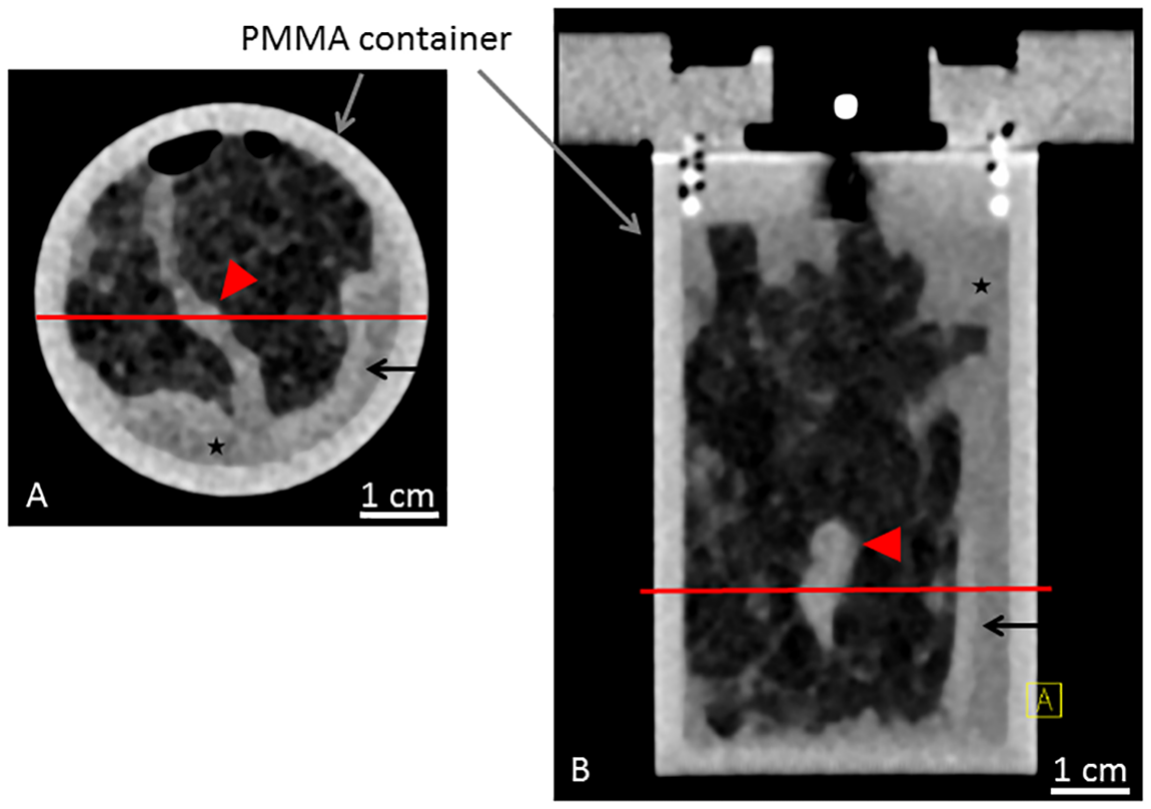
Clinical CT scan of sample B. Clinical CT scan of sample B in the sample holder with a standard clinical whole body CT scanner (Somatom Definition Flash, Siemens, Germany). Axial (A) and coronal (B) view. Red line in (A) and (B) reference for the respective imaging position. Asterisk indicating formaldehyde surrounding the sample. Red arrowheads indicating tumor. Arrows indicating skin.
